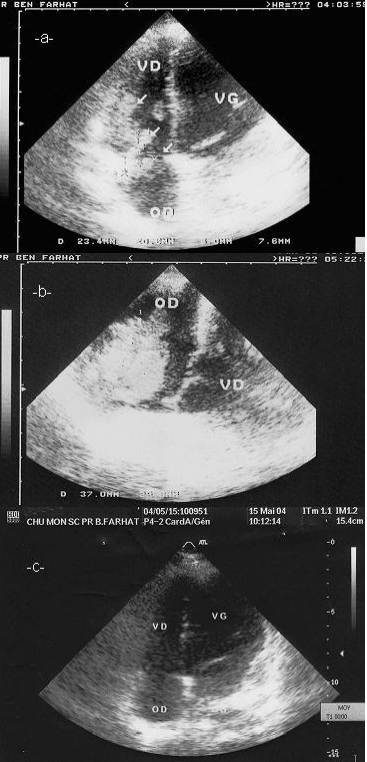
Transthoracic echocardiography: in apical four chamber view. (VD: right ventricle, VG: left ventricle, OD: right atrium, OG: left atrium) image of one thrombus in the right atrium, and two thrombi in the right ventricle (a), transoesophageal echocardiography showing a large thrombus (b), after treatment, complete resolution of the thrombus (c).
